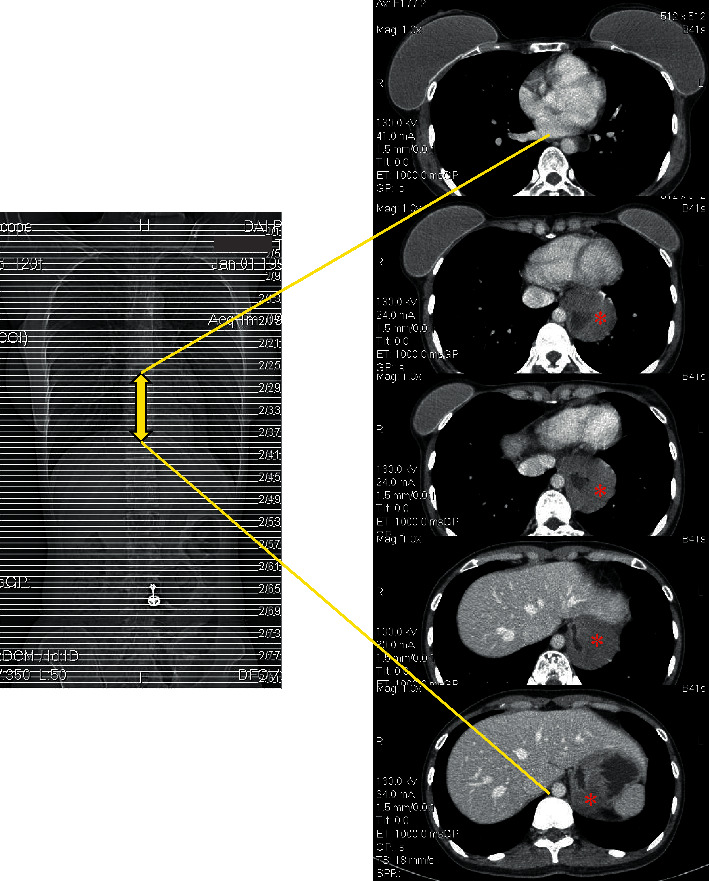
Uneven thickening of the distal esophagus and cardia on the CT scan, measuring about 8 cm in length and 3.5 cm in diameter. The asterisks mark the tumor mass.
